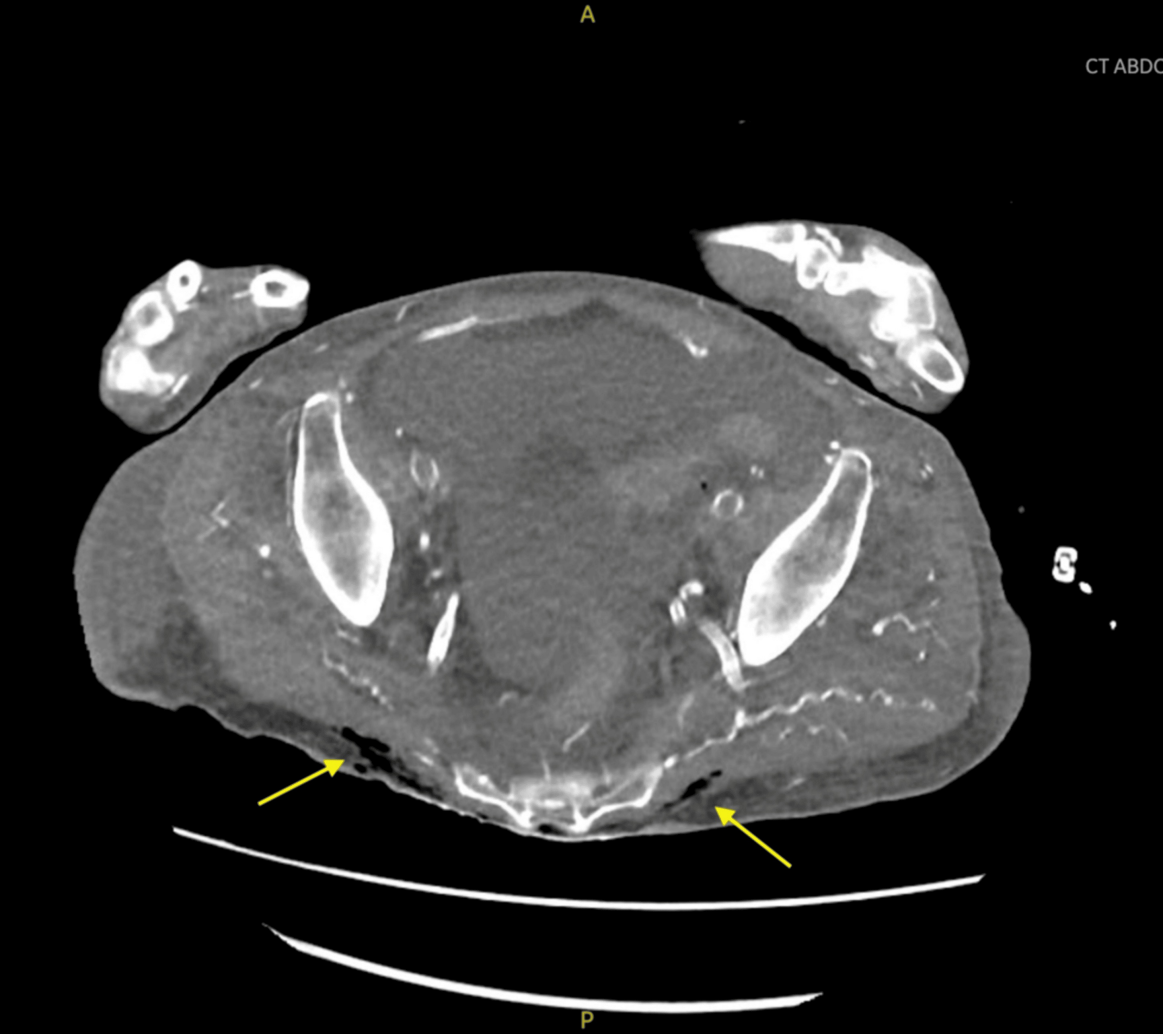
CT abdomen and pelvis without contrast showing subcutaneous gas along fascial planes in sacrum, coccyx, and right hip in a patient with decubitus ulcer
